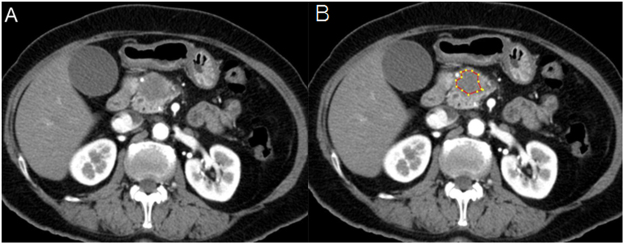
An example of quantitative texture analysis of the pancreas head cancer. (A) Axial pancreatic phase CT scan shows a low attenuating mass in the pancreas head. (B) ROI was drawn excluding fat or air densities for texture analysis.
